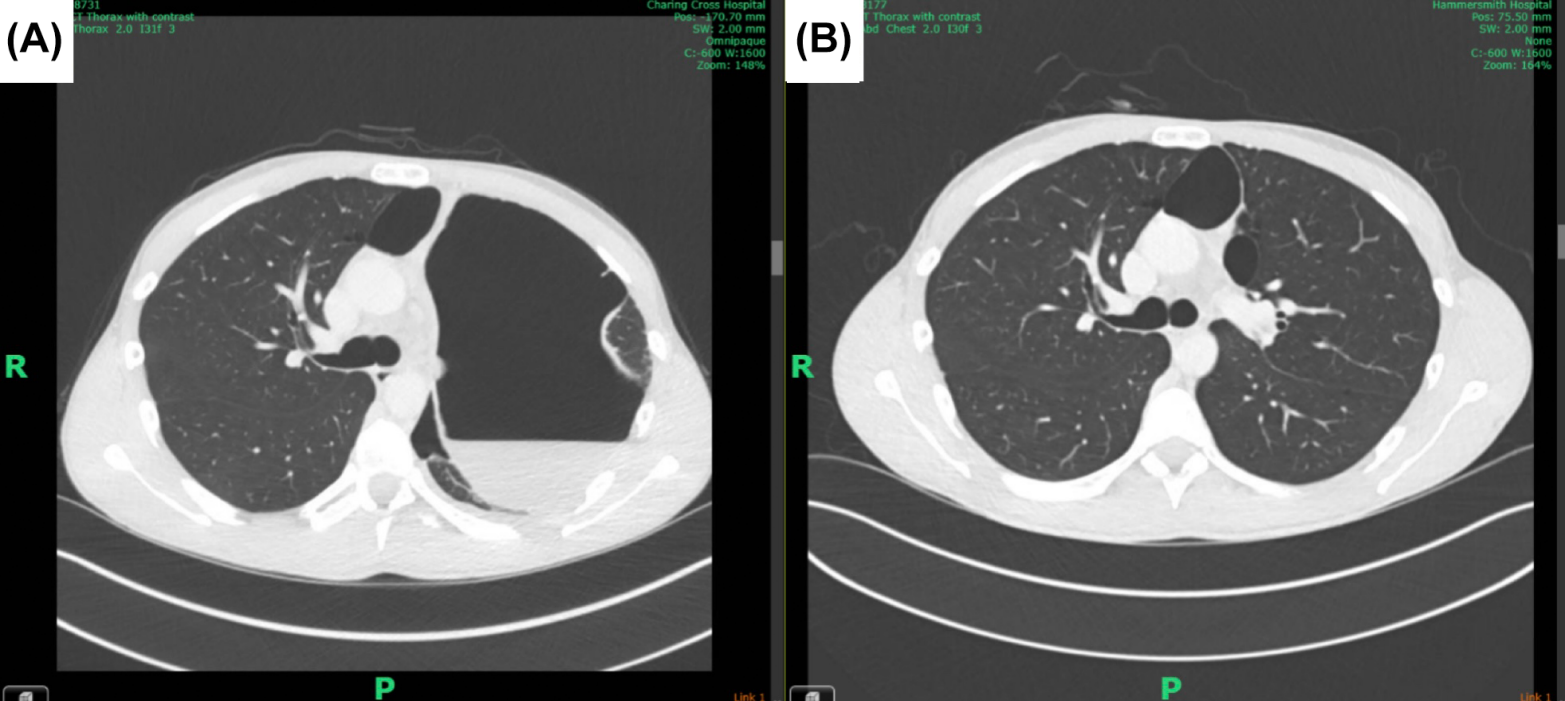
Side‐by‐side comparison of chest computed tomography on admission (A) and at 9 months (B) revealed the spontaneous resolution of the giant bulla in the left upper lobe.
